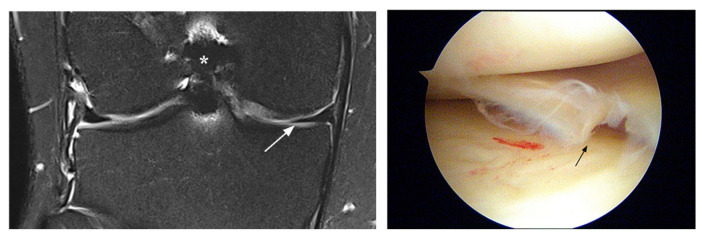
Unavoidable false-negative MRI diagnosis (patient 1). Coronal MRI shows normal appearance of the posterior horn of the medial meniscus (arrow), whereas the arthroscopic image indicates degenerative fraying with tear (arrow). Note status after ACL reconstruction (*).
